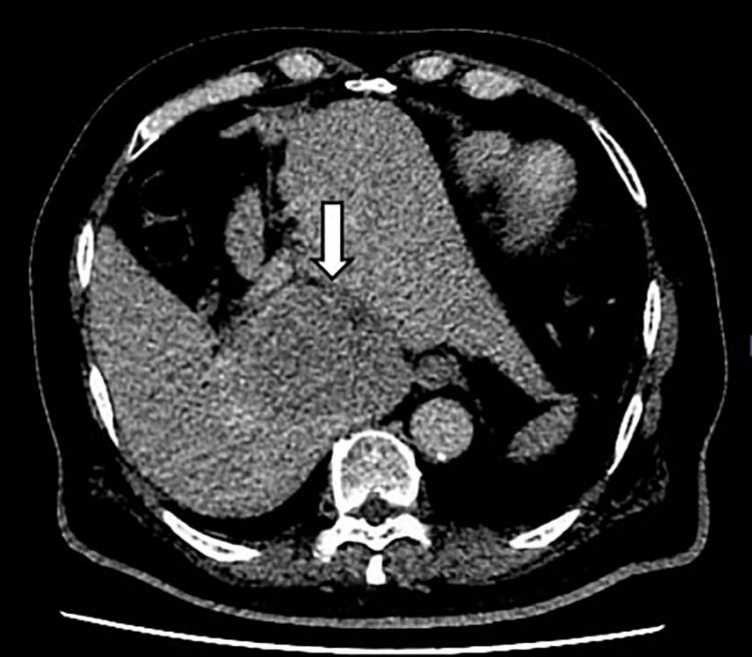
CT scan axial section delayed phase showing a 3.2 × 3.0-cm lesion (arrow) with early washout in Segment I of liver, consistent with hepatocellular carcinoma.
